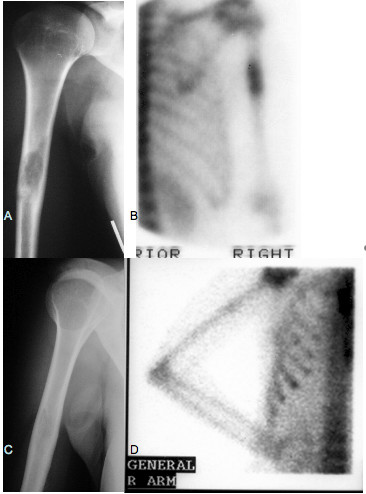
(A) Radiography and (B) bone scan of the right humerus at initial diagnosis showing Langerhans cell histiocytosis localization on the one-third medium which resolved (C and D) at the time of pulmonary manifestation.
